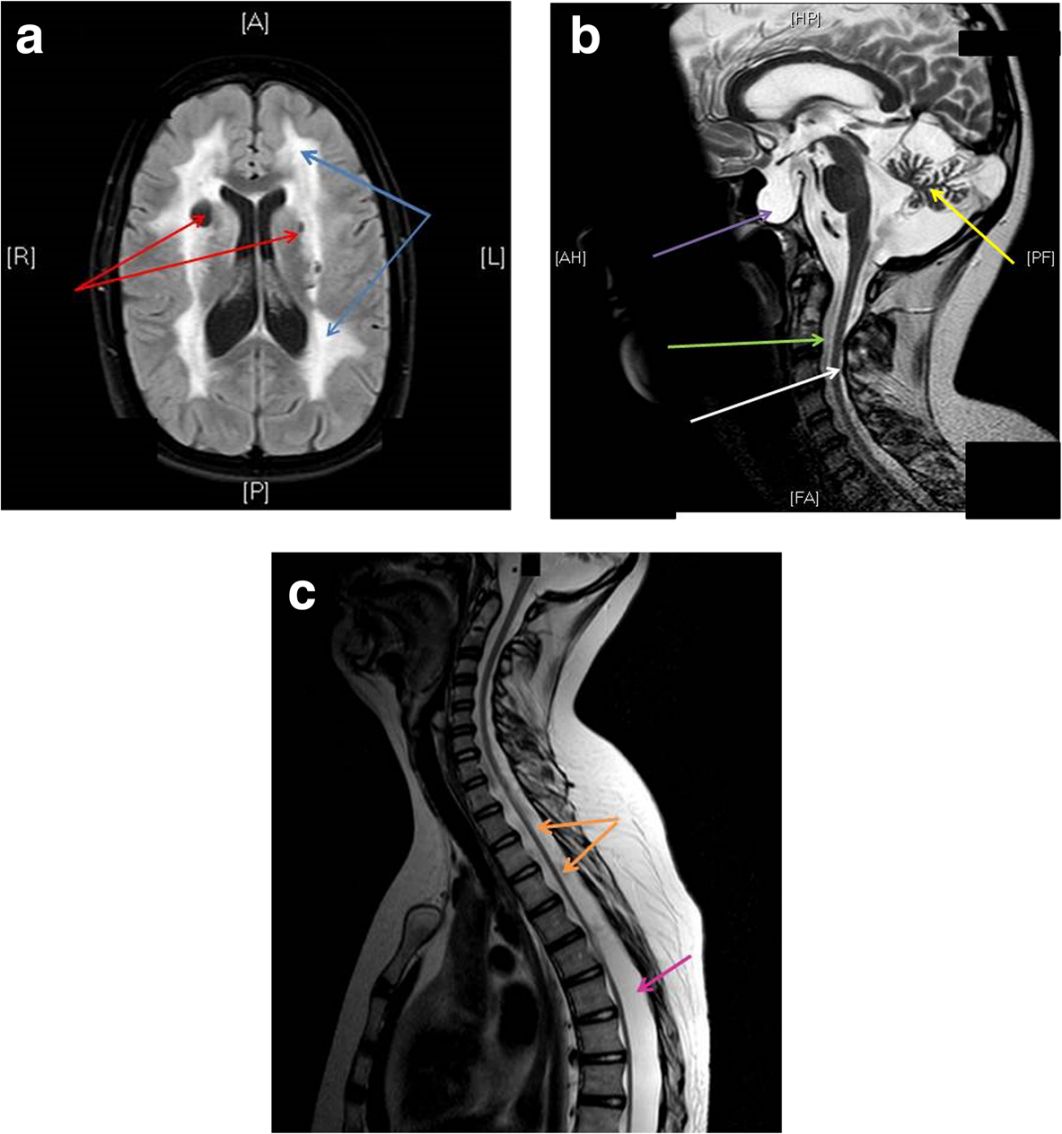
a MRI t2 FLAIR (transversal). Blue arrows: Confluent hyperintense signal of unknown origin engaging most of the white matter. Red arrows: Cysts in the white matter with the same signal as CSF. b MRI t2 (sagital). Purple arrow: Emty sella tursica partially filled with dura. Hypophysis compressed in dorsal direction. Green arrow: Dural ectasia. White arrow: Medullar atrophy. Yellow arrow: Cerebellar atrophy. c MRI t2 (sagittal). Orange arrow: Medullar atrophy. Whole medulla is affected. Pink arrow: Dural ectasia along the whole length of the medulla
